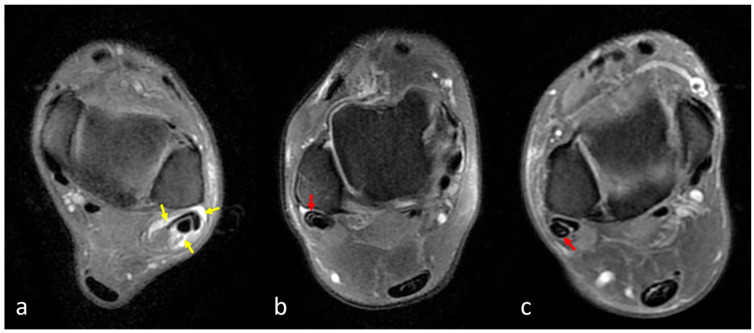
(a) Axial fat-suppressed proton density–weighted fast spin echo (FSE) MRI demonstrating peroneal tenosynovitis. A high-signal-intensity rim is observed surrounding the peroneal tendons (yellow arrows), indicating abnormal fluid accumulation within the tendon sheath. The fluid thickness exceeds 3 mm at its widest point, consistent with the diagnosis of tenosynovitis. (b,c) Axial fat-suppressed proton density–weighted fast spin echo (FSE) MR images demonstrating peroneal tendinopathy. Increased intratendinous signal intensity of the peroneus brevis (red arrow) (b) and longus tendons (c) (red arrow) is seen without fiber discontinuity. The findings are consistent with peroneal tendinopathy (tendinitis).
